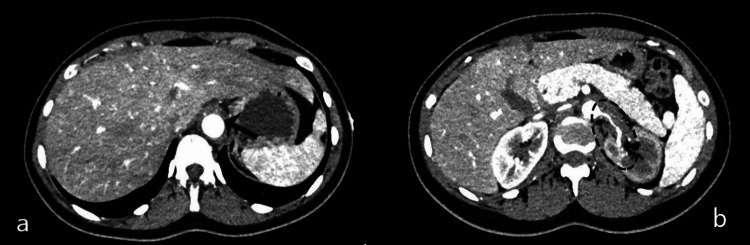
(a) Axial post-contrast CT angiogram of the abdomen showing mural wall thickening of the aorta (black solid arrow). (b) Axial post-contrast CT angiogram showing significant stenosis of the left renal artery (black dashed ellipse). CT: computed tomography
